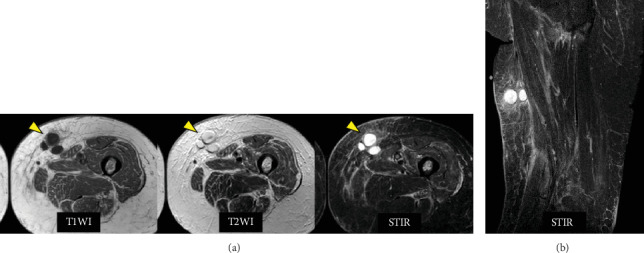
MRI images of femoral subcutaneous cysts. MRI of the left femur: (a) axial view and (b) coronal view. The subcutaneous cyst was located just beneath the sartorius muscle, approximately 18 cm distally from the pubic symphysis. The subcutaneous multifocal cyst (yellow arrow) showed diffuse T1, T2, and STIR hyperintensities. There was no inflammatory spillover around the cysts. This indicated that the mass was a nonmalignant tumor surrounded by a capsule.
